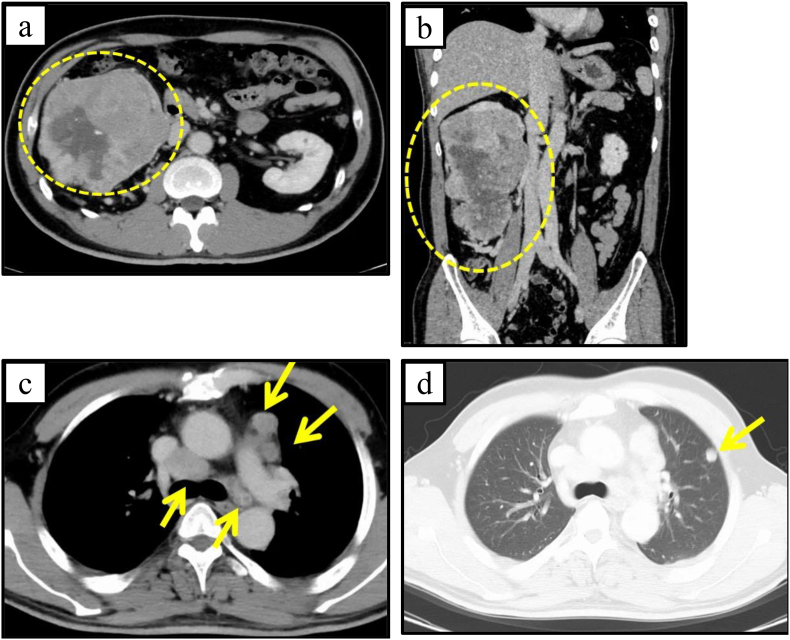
CT findings at the first visit. (a,b) Axial and coronal images show a right renal mass, strongly enhanced in the arterial phase due to hypervascularization and washed-out in the nephrogenic phase. (c) Hilar lymph nodes are enlarged. (d) Multiple lung metastases.
